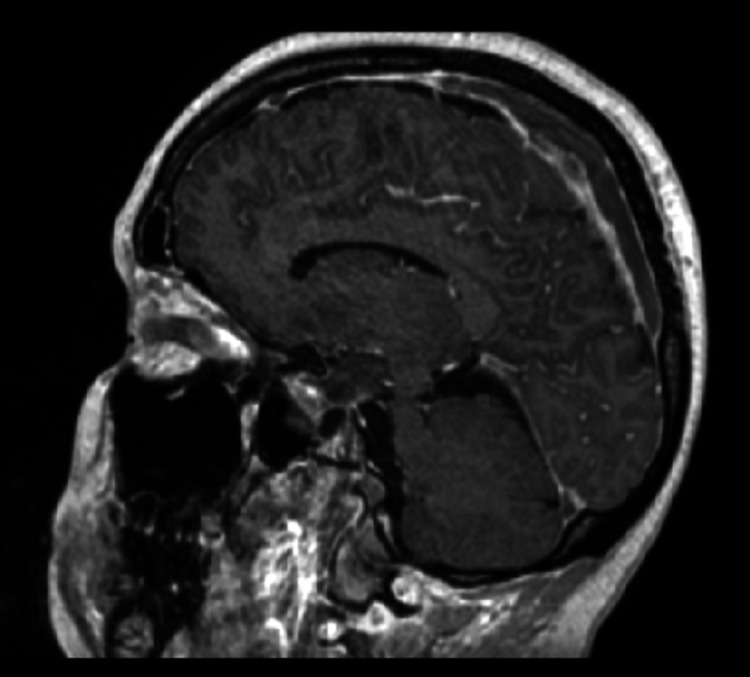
MRI of the brain: acute/early subacute ischemic infarct involving the left frontal, parietal and occipital lobes with effacement of the sulci. No definite hemorrhagic transformation is seen. There is extensive thrombosis involving the dural sinuses.
